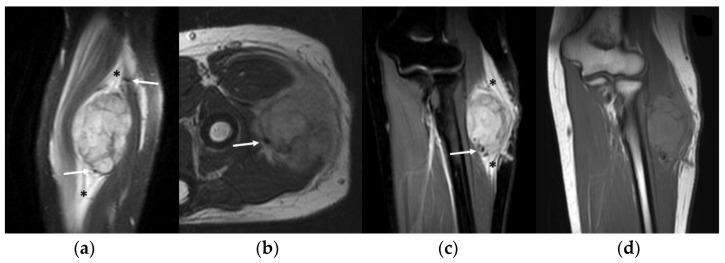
MRI of a 16-year-old female with alveolar soft part sarcoma of the forearm. Note the intra-/peritumoral flow voids on fluid-sensitive sequences can be noted as well (arrows). (a) Sagittal and (c) coronal Proton Density fat-saturated images demonstrate a moderately hyperintense neoplasm with diffuse peritumoral edema (asterisks); (b) axial T2-WI; (d) coronal T1-WI shows an inhomogeneous slightly hyperintense mass.
